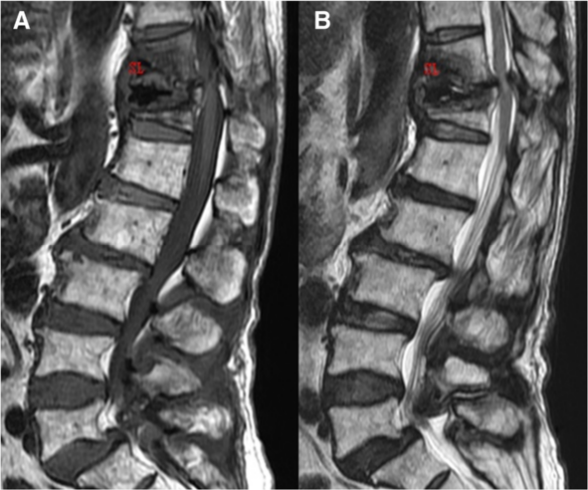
Sagittal MRI images. Sagittal T1-weighted MRI (A) showed T11 and T12 fracture with bone marrow edema. Sagittal T2-weighted MRI (B) revealed fluid accumulation surrounding T12 bone cement. Both sagittal T1- and T2-weighted MRI demonstrated T11 and T12 severe stenosis due to spinal instability.
